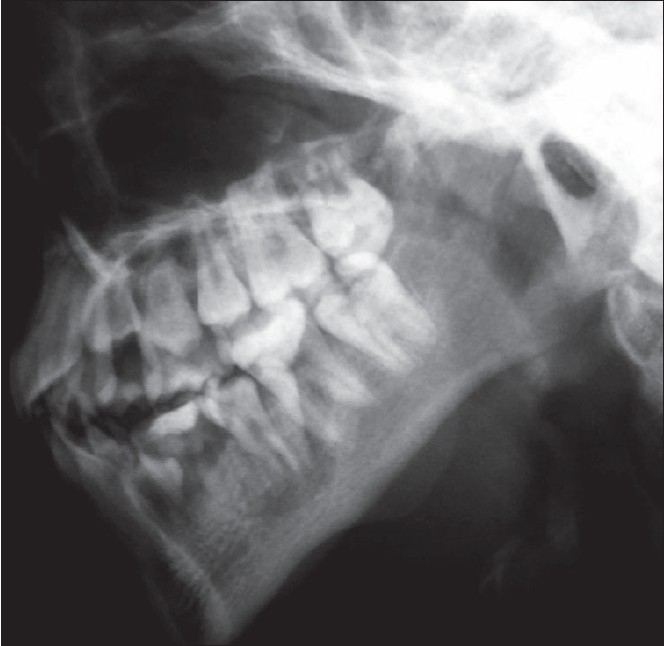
Lateral radiograph of the mandible shows small mandible with small ascending ramus and infantile obtuse angle
